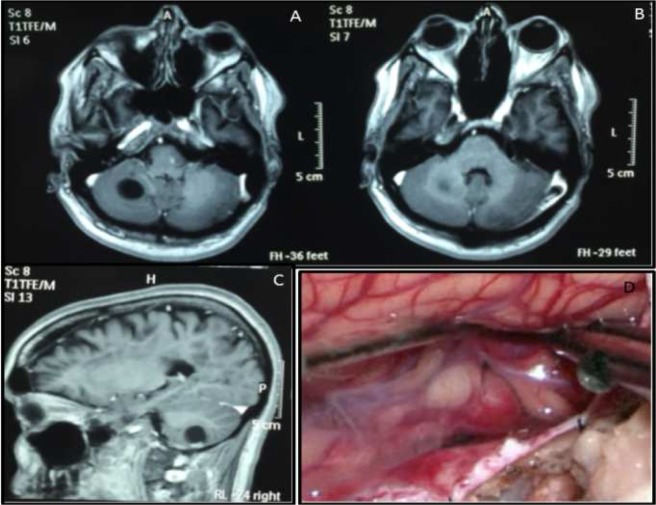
Magnetic resonance imaging (MRI) of a cystic formation on the cerebellum (A, B and C); detail of surgical resection of the tumor (D), later confirmed to be an hemangioblastoma
